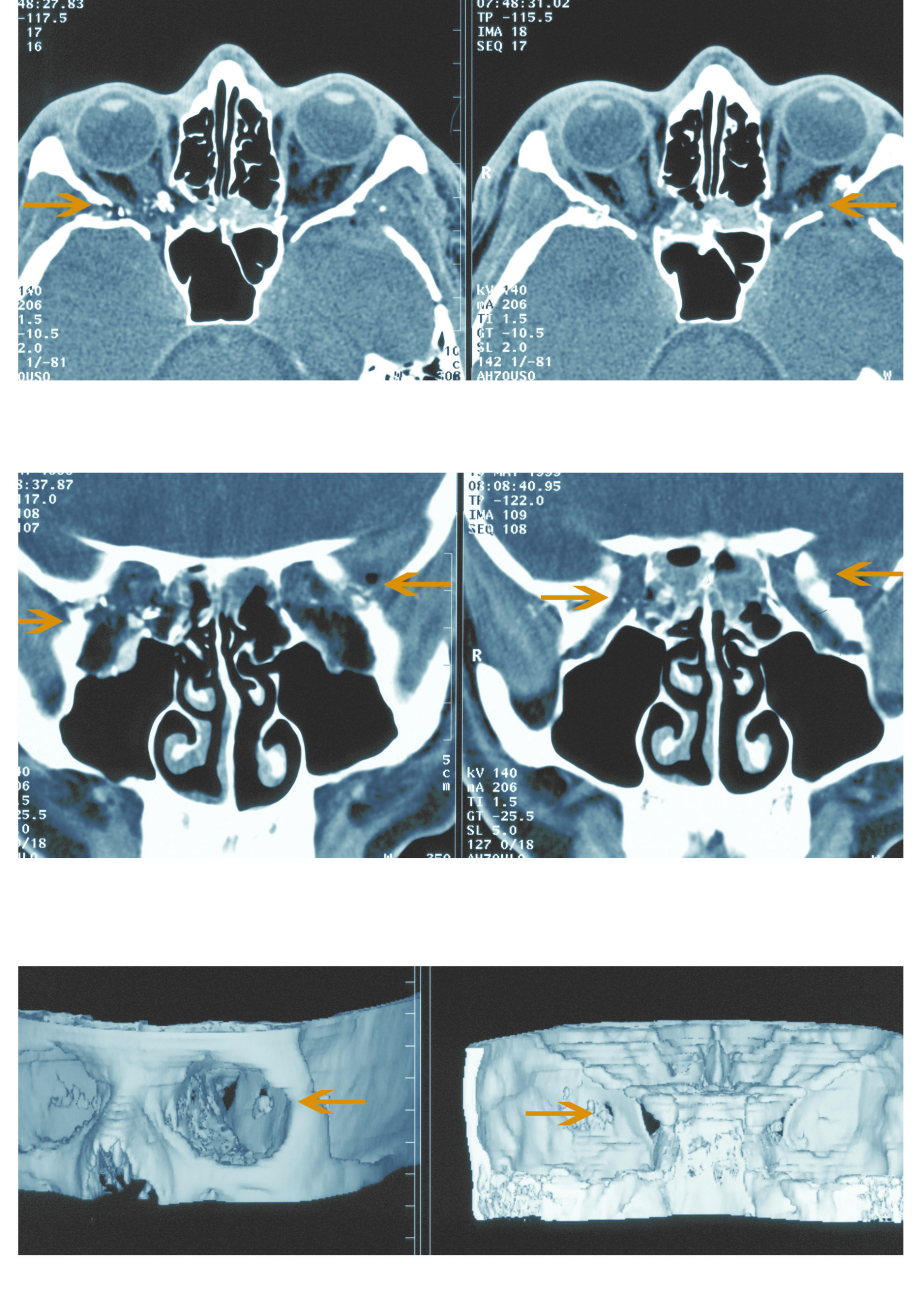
Entrance and exit wounds (see arrows) revealed by computerized tomography (CT) scans (axial and coronal views) in conjunction with three-dimensional (3D) orbital reconstruction (anterior and posterior views).
